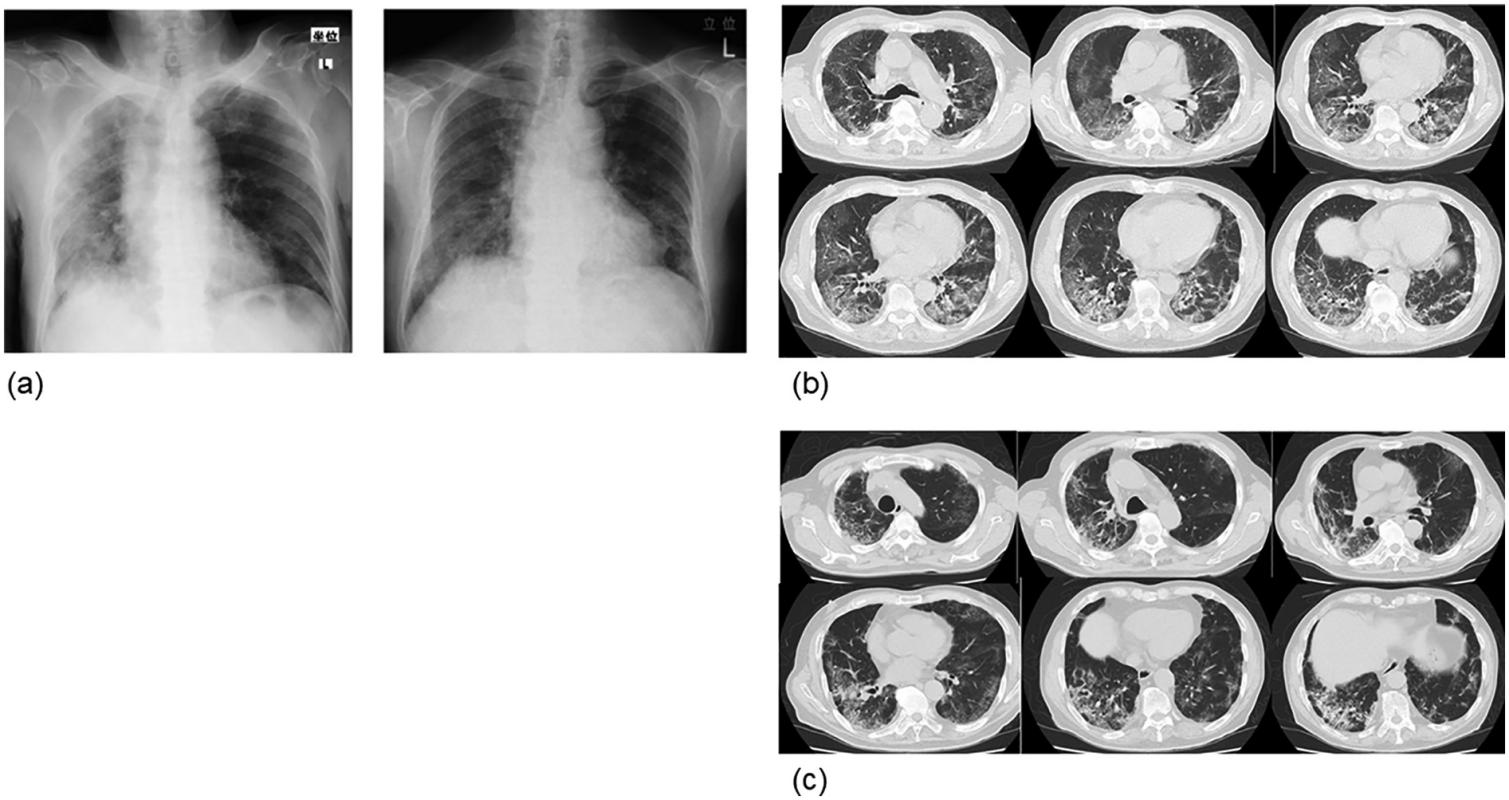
Chest radiograph and computed tomography image (case 1). (a) The left is at the time of onset and the right is at the time of admission to our hospital (1 month after onset). Improvement in interstitial inflammatory changes in the right lung was observed, but inflammatory changes persisted in the right lung. (b) At the time of onset. Frosted glass-like shadows were observed around the margin, and interstitial inflammatory changes were observed on both dorsal sides. (c) One month after admission to our hospital (2 months after onset). The left interstitial inflammation improved, as seen in the chest radiograph, but the interstitial inflammatory changes on both sides, mainly on the dorsal side of the right lung, remained.
